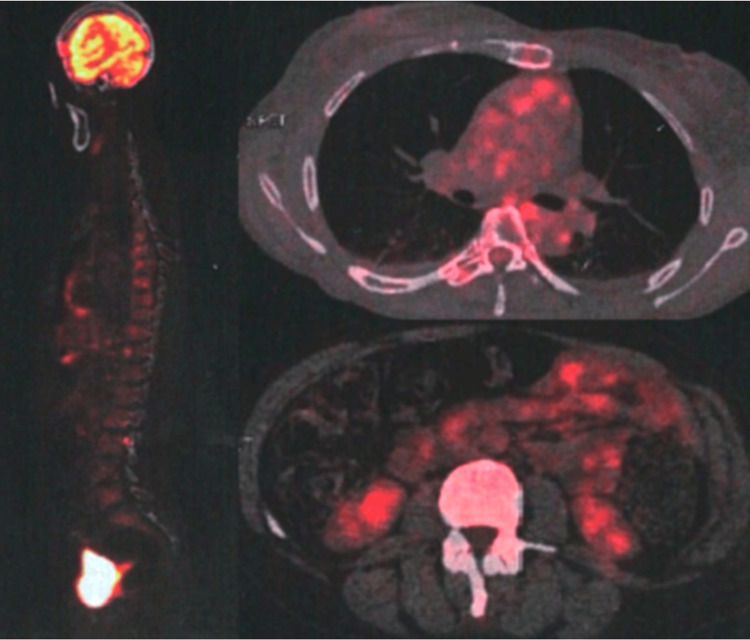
Positron emission tomography (PET) with no hypermetabolic fluorodeoxyglucose (FDG)-avid lesions suggestive of malignancy
